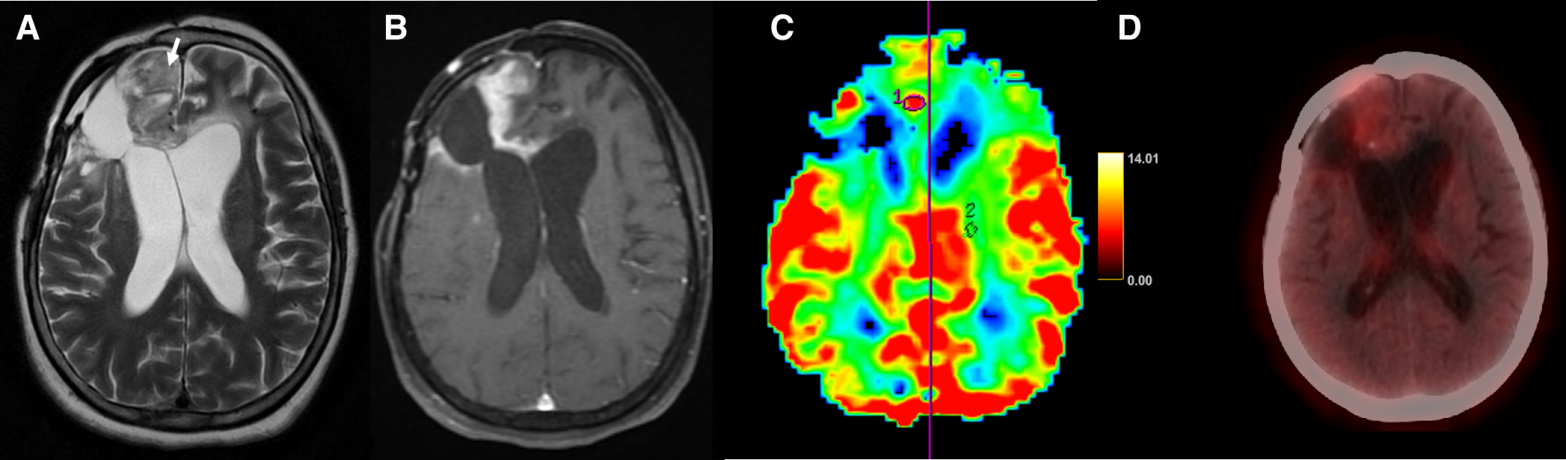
Axial T2-weighted (A), T1 + Gd (B), perfusion map (C) and FET-PET (D) images in a case of glioblastoma shows T2 intermediate areas (arrow) in the right frontal lobe. Thick peripheral enhancement (B) and hyperperfusion (C) is seen in ROI1 placed in the enhancing component and ROI2 in the normal white matter. FET-PET (D) shows no significant uptake (T/W ratio: 2.2) in the same area. This was a case of radionecrosis, with false positive results on MRI.
